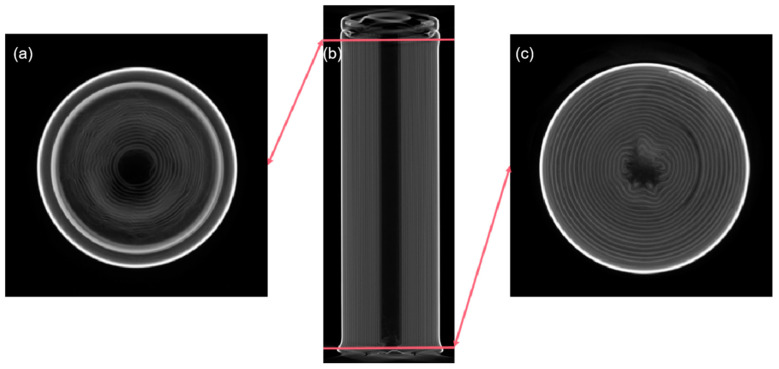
CT inspections after axial impact under 11.6 m/s impact: (a) cross-section of the top section; (b) side view; (c) cross-section of the bottom section.
